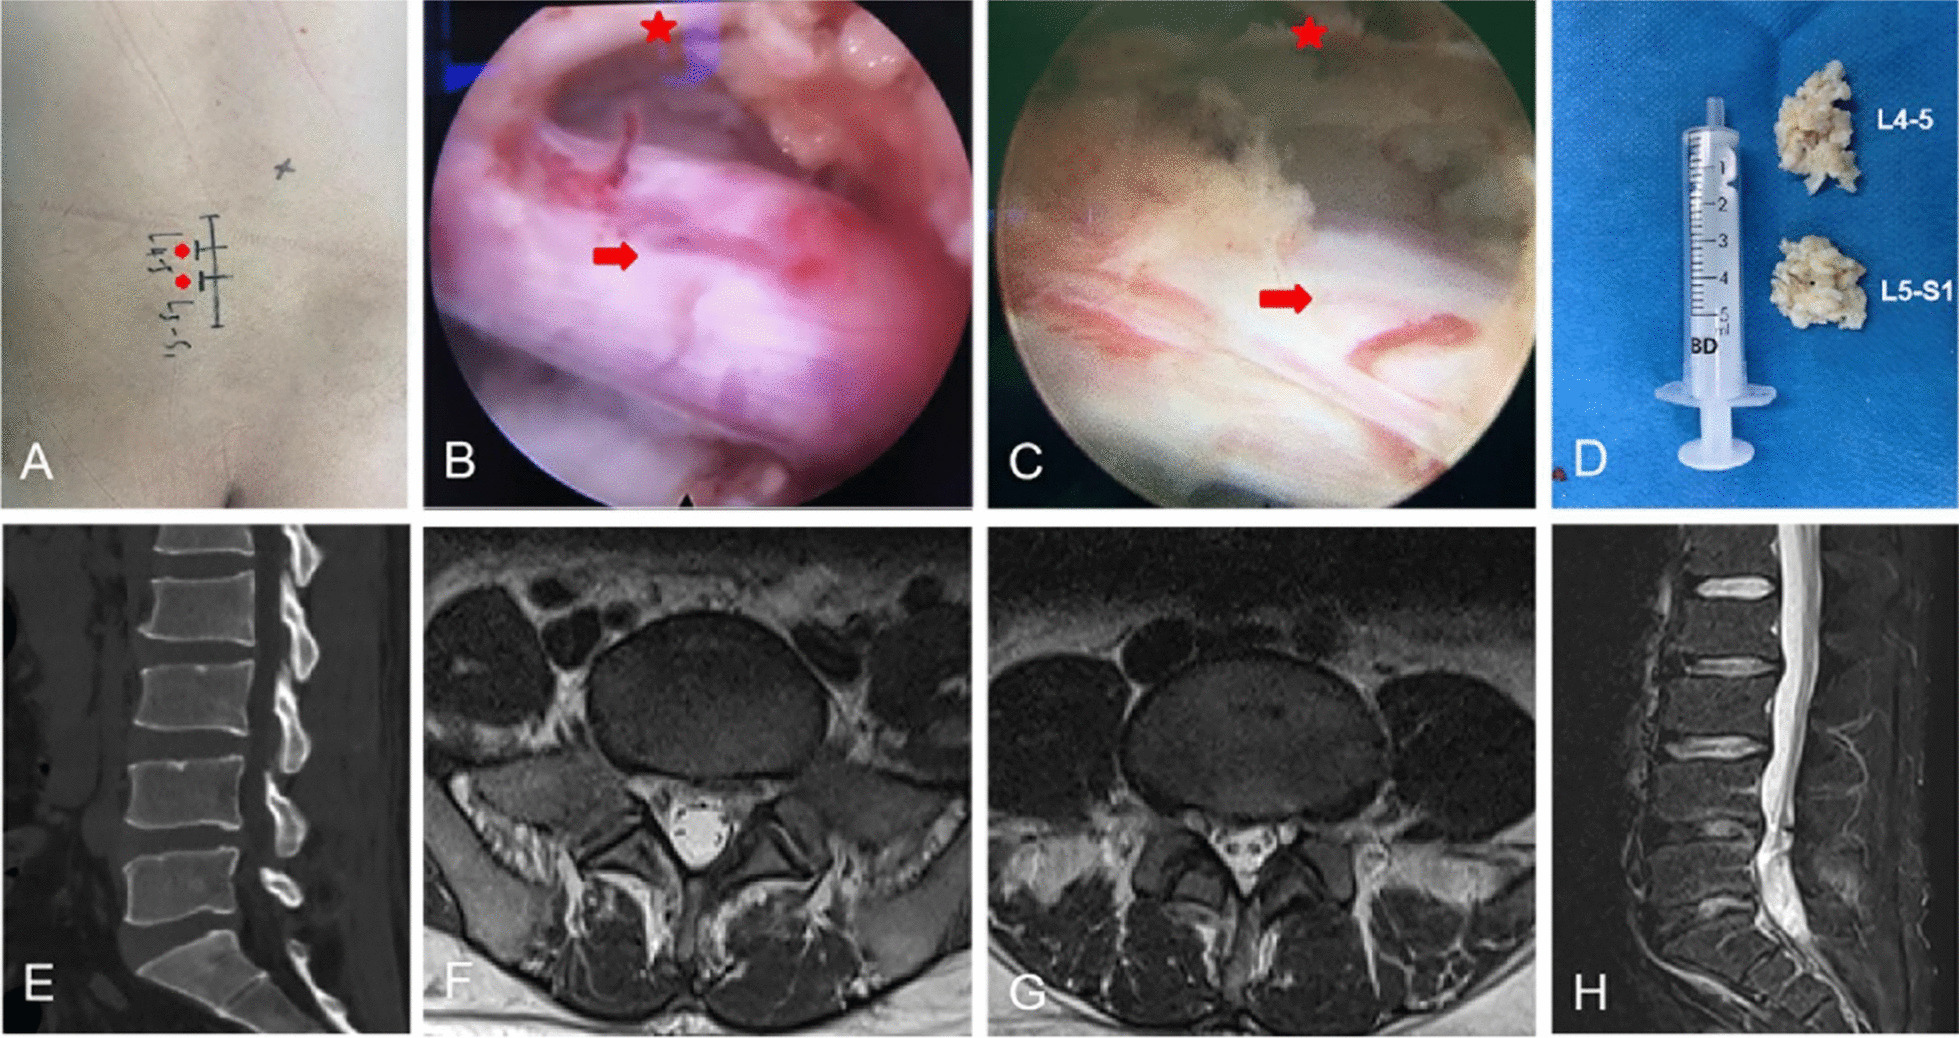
Intraoperative images and postoperative radiological examination. A: Preoperative incision planning (red arrow). B: Intraoperative view of the interlaminar access with L5 nerve root (arrow) and dural sac (star). C: Intraoperative view of the interlaminar access with S1 nerve root (arrow) and dural sac (star). D: Disk pulposus. E–H: CT and MRI at 3 months postoperatively showing good decompression of nerve root and dura
